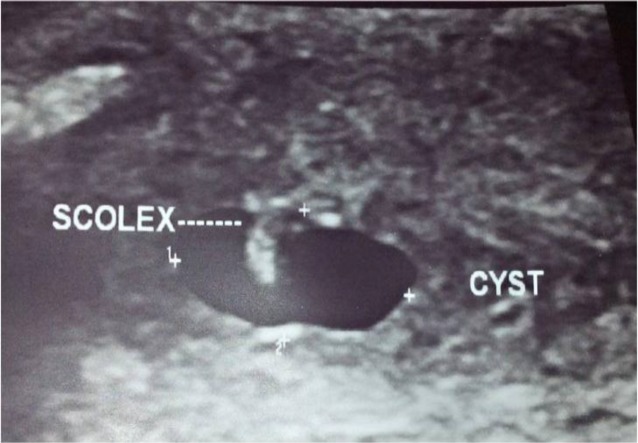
Ultrasound tracing of neck showing the features of a definite cystic lesion with a scolex within it
